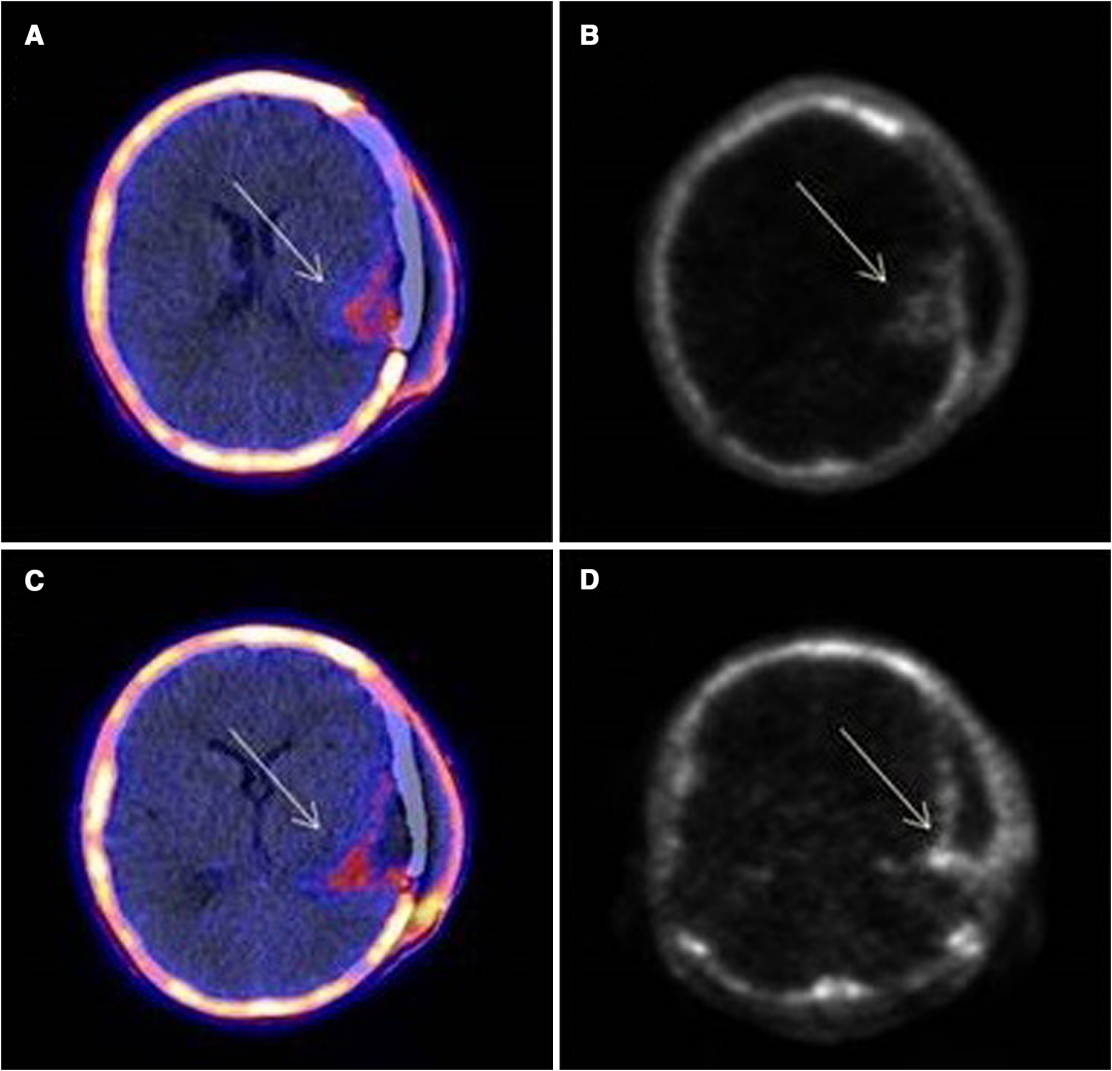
PET/CT (a, c) and PET (b, d) images of glioblastoma multiforme: increased ^18^F-FLT uptake (*arrows*) at parts of the borders of surgical excision (SUV_max_ 1.29, tumor-to-background ratio 10.75). CT* computed tomography, *FLT* fluorothymidine, *PET* positron emission tomography, *SUV* standardized uptake value
